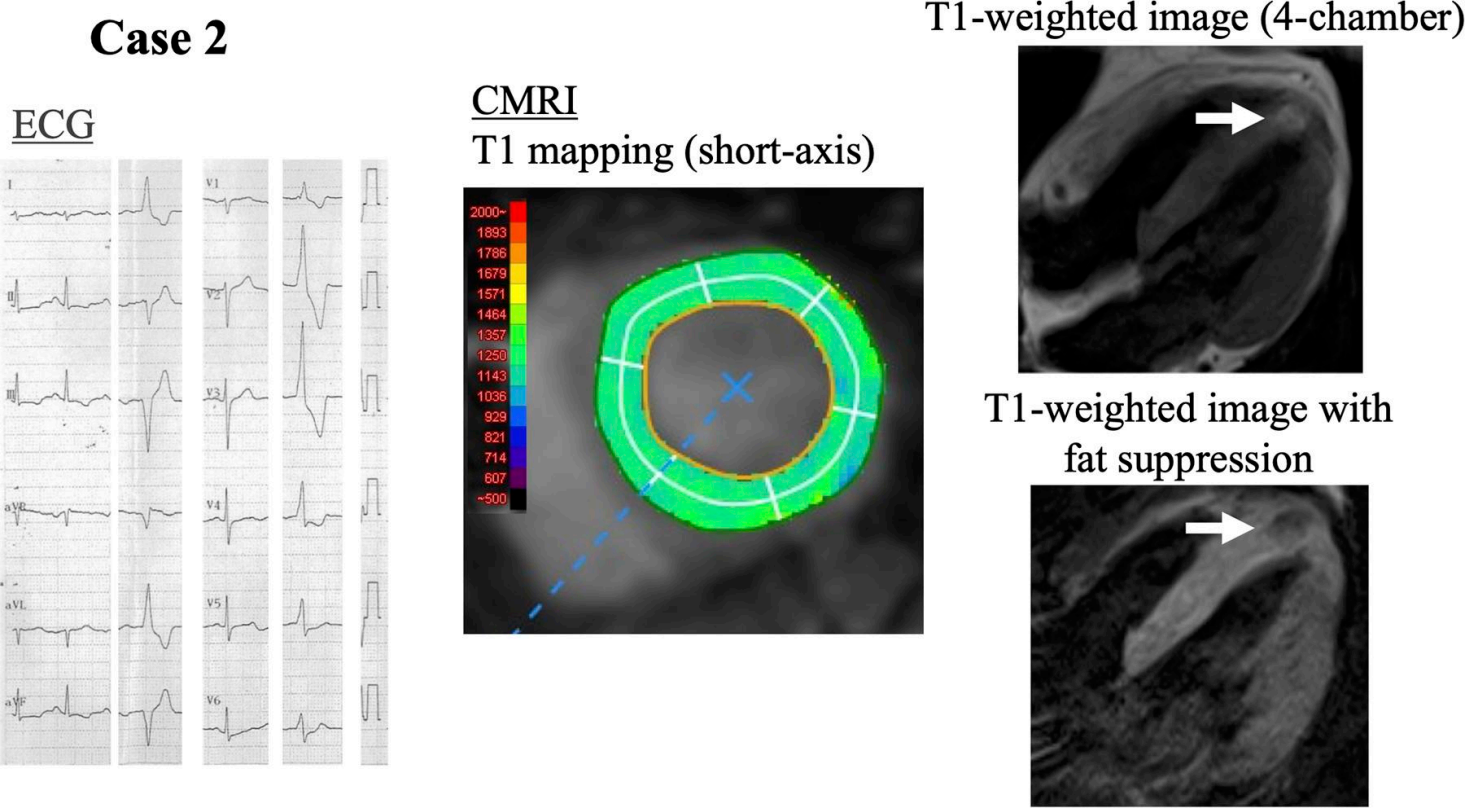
A 40-year-old woman with suspected ALVC. Case 2: a 40-year-old woman with monomorphic PVCs that originated from the left ventricular inferior wall. No LGE on CMRI, but high native T1 values (maximum 1,302 ms). T1-weighted image with fat suppression shows intramyocardial fat signal of suspected ALVC (white arrow). PVCs, premature ventricular contractions; LGE, late gadolinium enhancement; CMRI, cardiac magnetic resonance imaging; ALVC, arrhythmogenic left ventricular cardiomyopathy.
